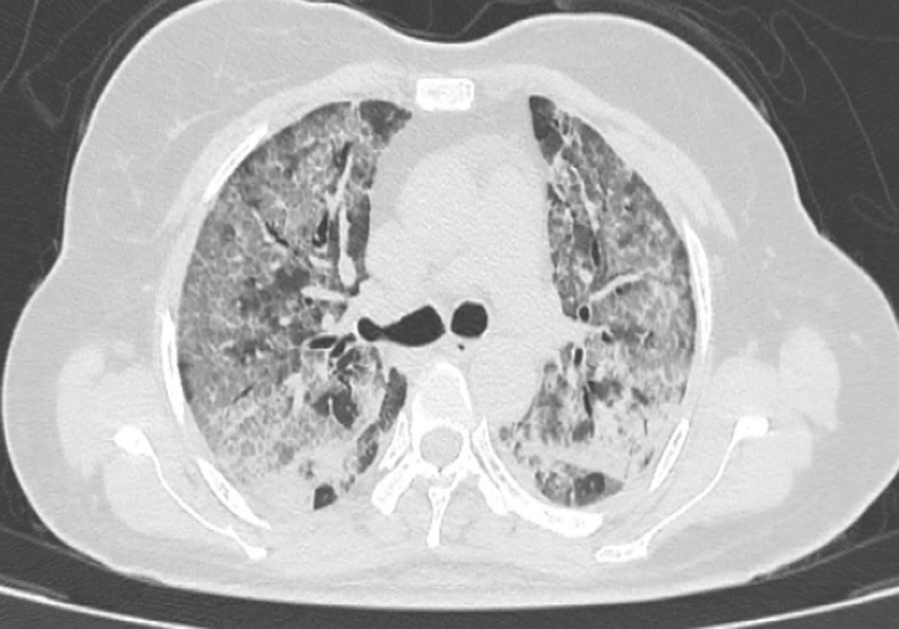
Axial CT image indicating severe disease severity (CTSS 20). Axial CT image shows extensive GGOs, crazy paving, and consolidation mainly in lower lobes bilaterally with CTSS 20
